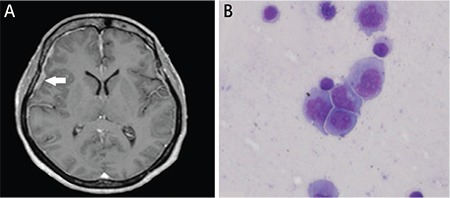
A) Contrast-enhanced magnetic resonance imaging of the brain showing patchy meningeal enhancement and thickening, suggestive of pachymeningitis. B) Cerebrospinal fluid cytology showing atypical lymphoid cells 2-3 times the size of normal lymphoid cells with prominent nucleoli.
